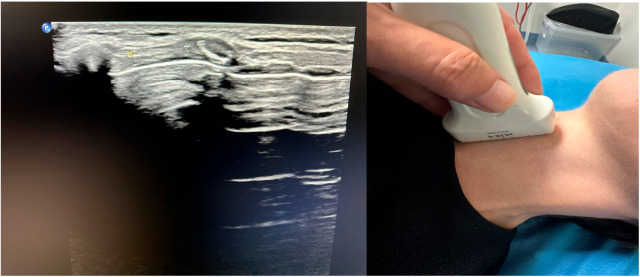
Ultrasonographic measurement of the cricothyroid membrane length (CTML): linear probe placed sagittally (right) and the corresponding sonographic view (left).
